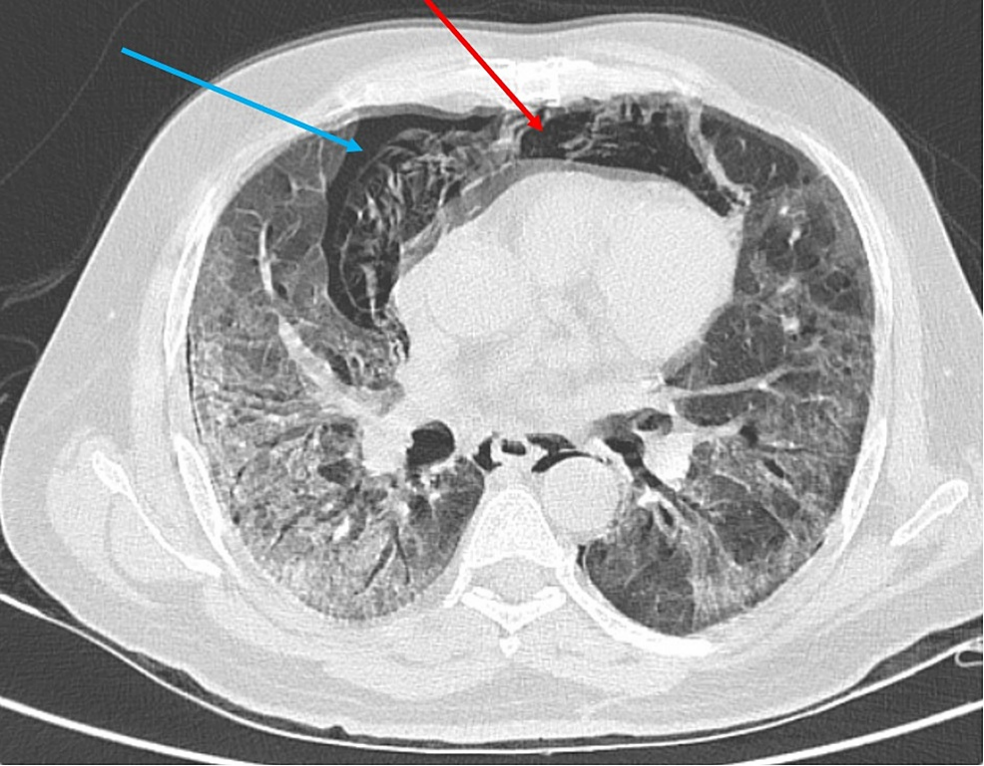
High-resolution computed tomography of the thorax-axial section showing pneumomediastinum (red arrow) and pneumothorax (blue arrow).
